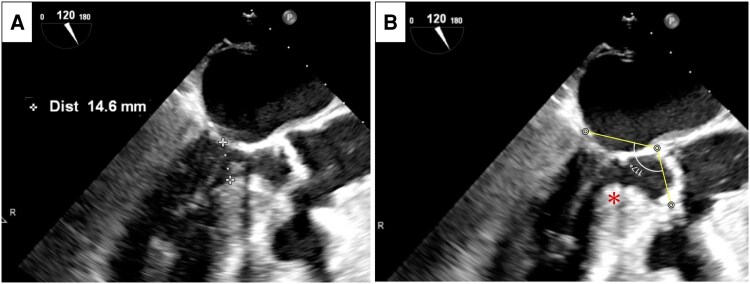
Morphologic risk factors for systolic anterior motion in our patient. (*A*) Transoesophageal echocardiography showing a short distance (Dist) between the coaptation point and septum (14.6 mm). (*B*) The aortomitral angle measured in the same view is narrow (117°). The basal interventricular septum has a sigmoid configuration (*).
